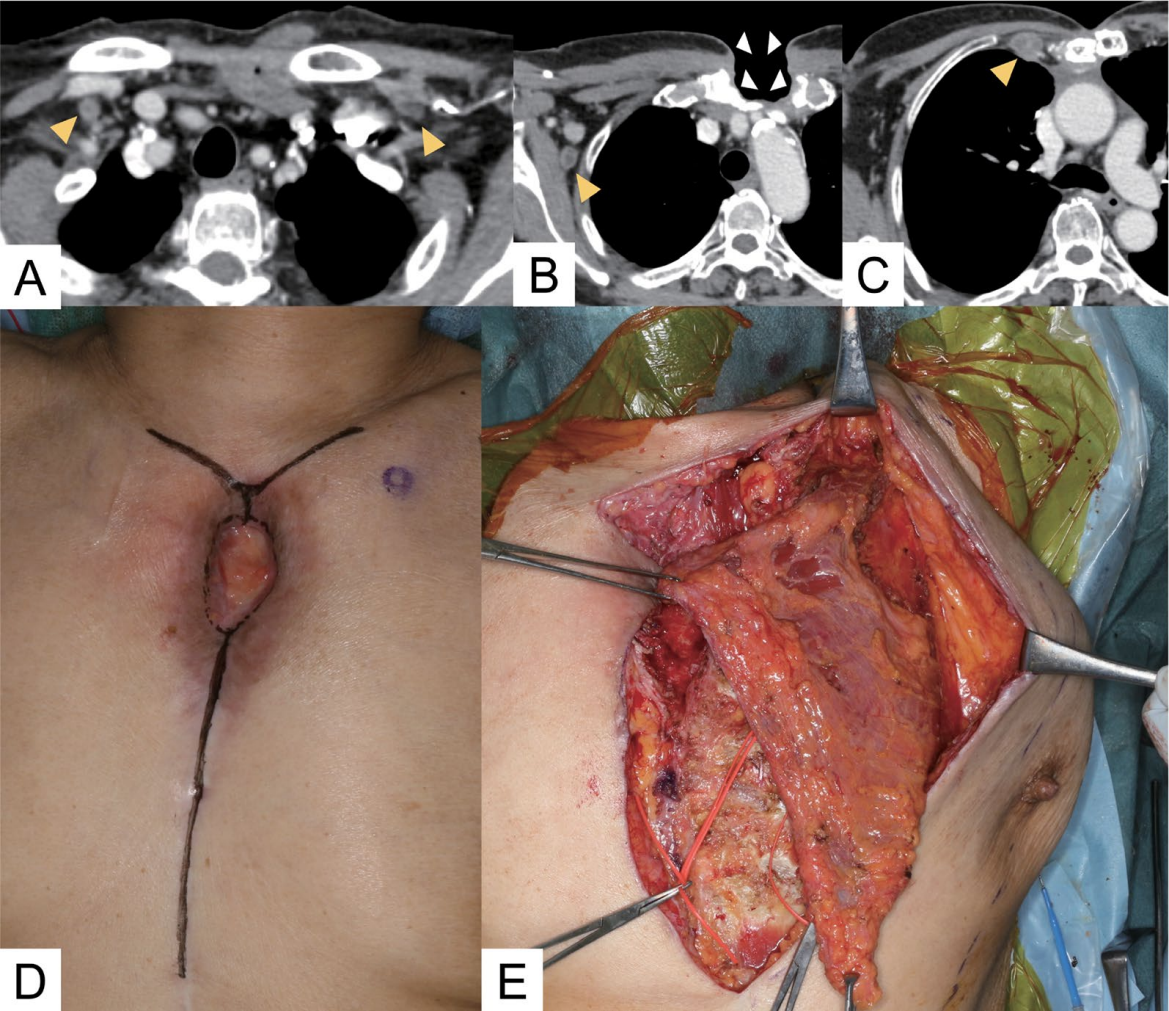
A–C CT shows lymphadenitis in bilateral supraclavicular, right axially, and parasternal lymph nodes (yellow arrowheads). The wound is left open (white arrowheads). D Appearance of the wound before wound closure. E Intraoperative findings. Left pectoralis major flap was used for wound closure to fill the space
